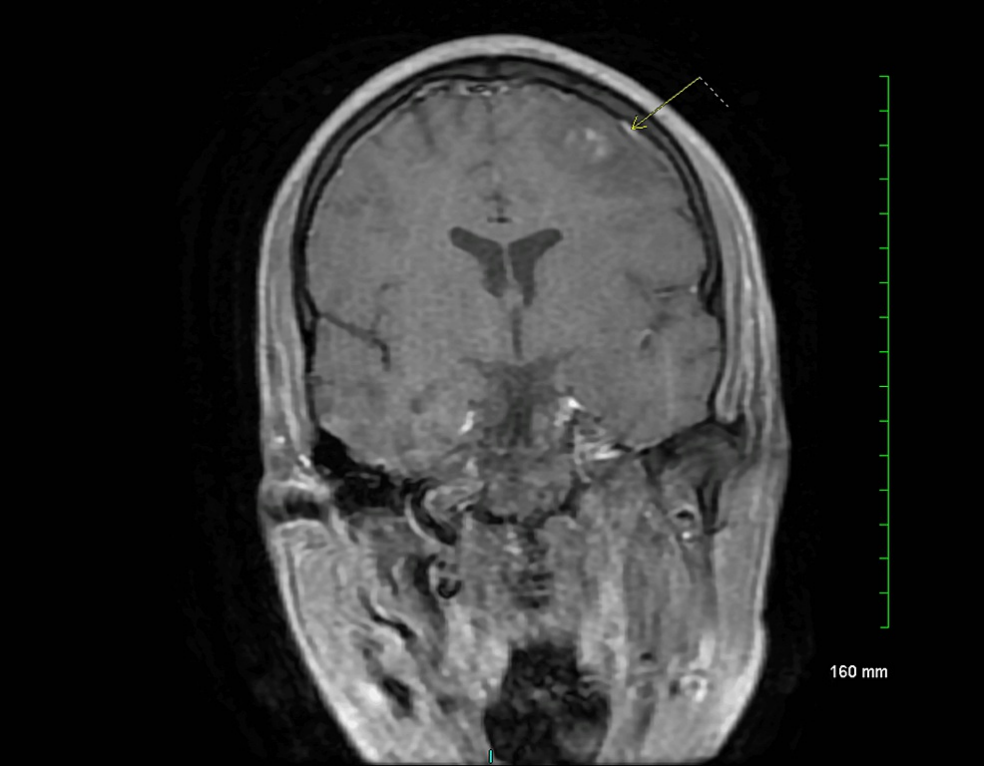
MRI of the brain showing acute disseminated encephalomyelitis (see arrow to identify the lesions)
